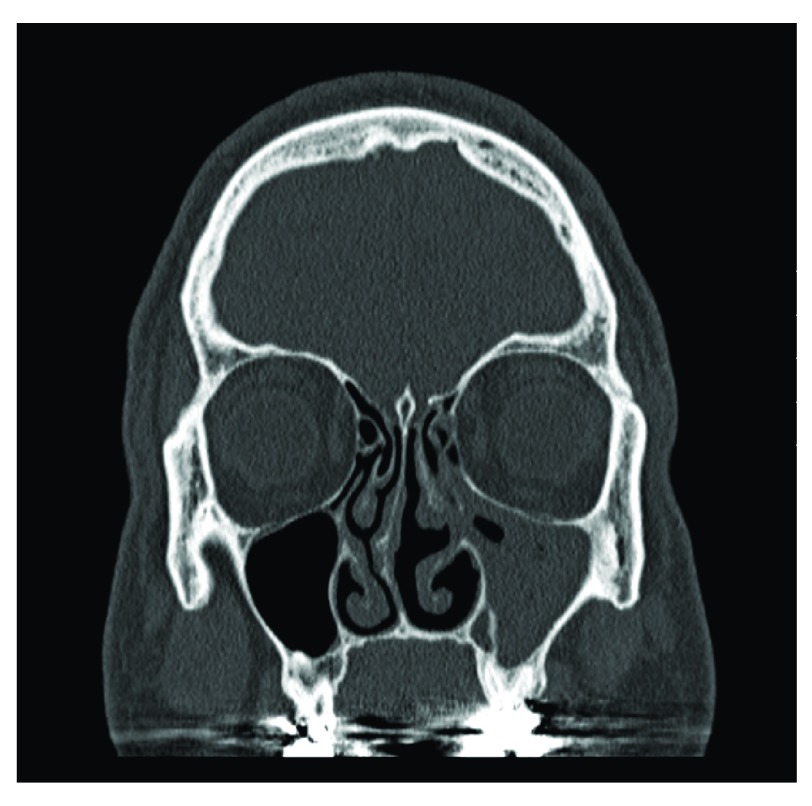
Chronic rhinosinusitis without nasal polyps (CRSsNP). Coronal CT scan of CRSsNP limited to the left maxillary and anterior ethmoid sinuses secondary to a point of obstruction within the left ostiomeatal complex.
